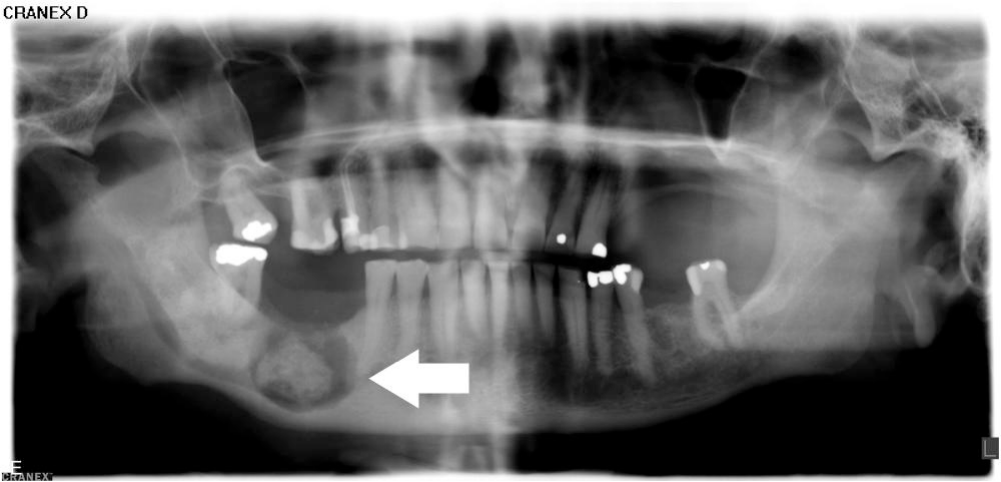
Panoramic x-ray of the jaws from 2023 showing an intraosseous lesion in the lower right mandible.
